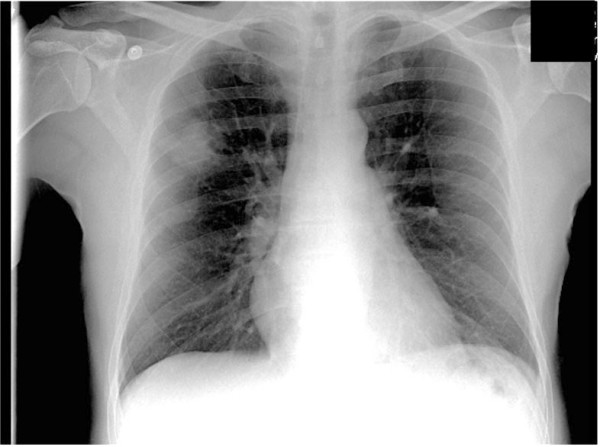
Chest radiograph showing shadowing in the right upper zone.
